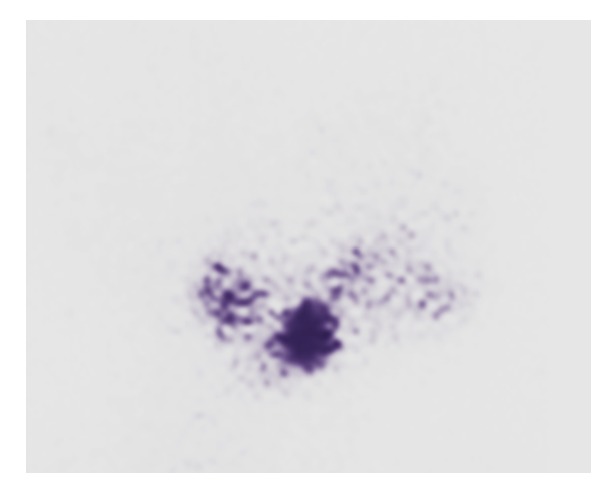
Posttherapeutic scintigraphy.
